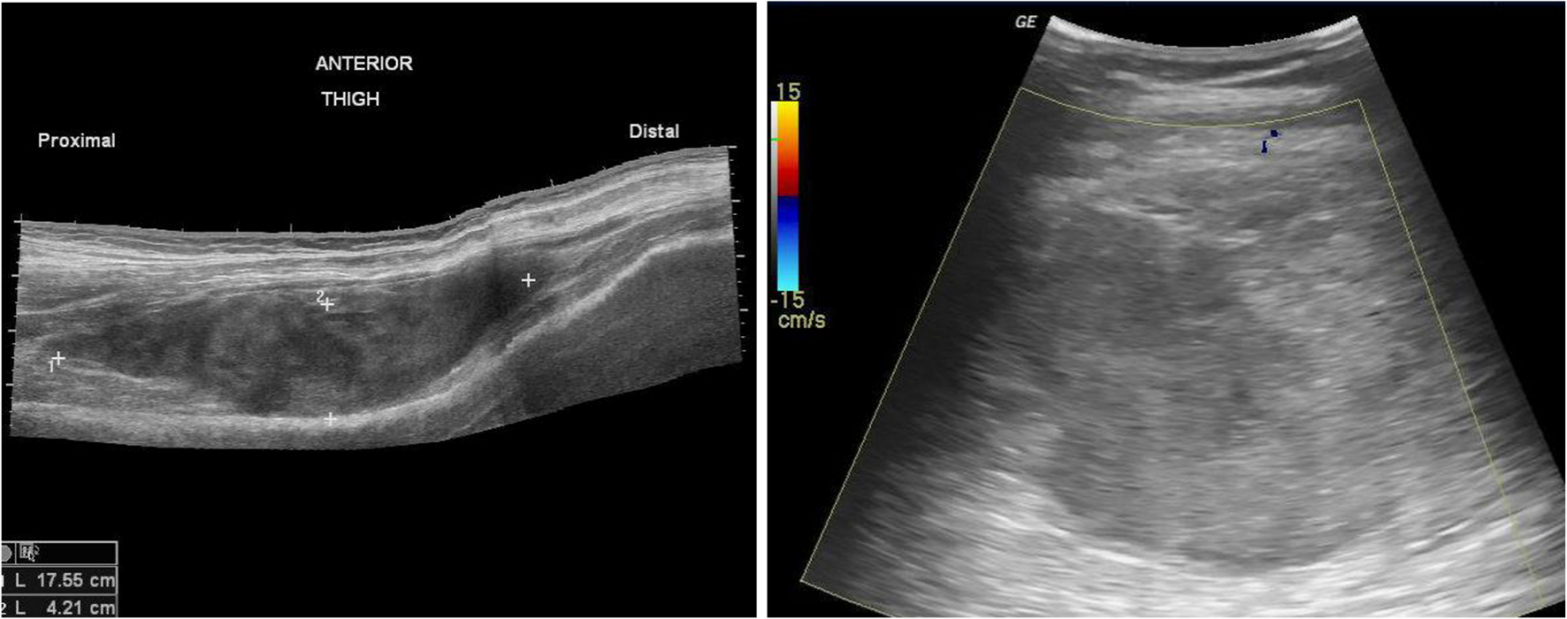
a Long axis view of the anterior thigh shows a well-defined area in the vastus intermedius muscle consistent with a hematoma. b Doppler short axis view of the lesion shows no vascularity. Case courtesy of Dr Maulik S Patel, Radiopaedia.org, rID: 24042
